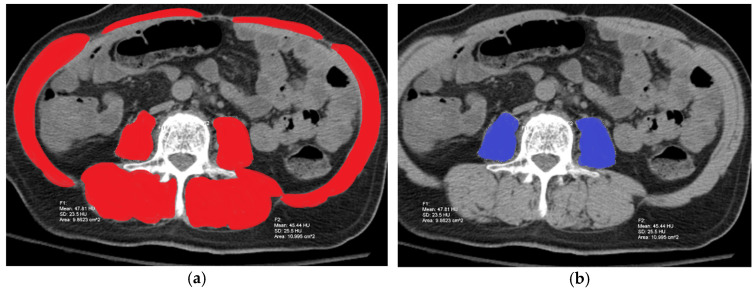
CT scan of a 68-year-old male patient at L3 demonstrating how sarcopenia is assessed based on measurement of SMI in red (a) as defined by cross-sectional areas of psoas, erector spinae, quadratus lumborum, transversus abdominis, rectus abdominis, and internal and external obliques, and measurement for psoas muscle area (PMA) in blue (b) as measured by the sum of the areas of the psoas alone.
